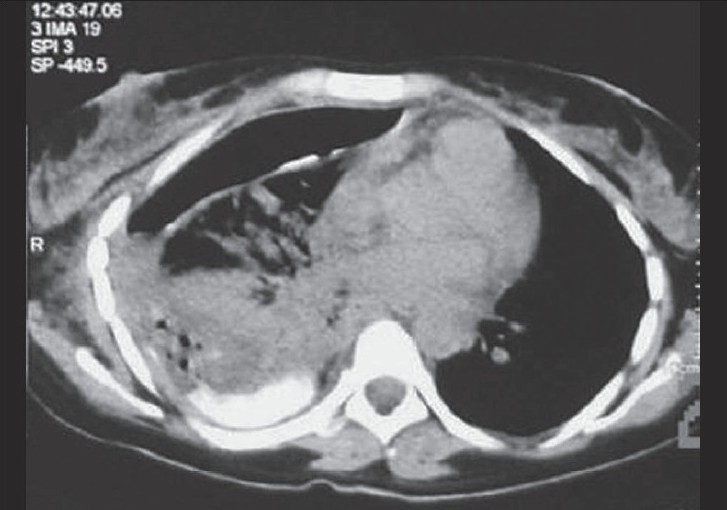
CT scan of the chest 1 day after barium enema shows barium layering along the posterior part of the right pleural cavity
